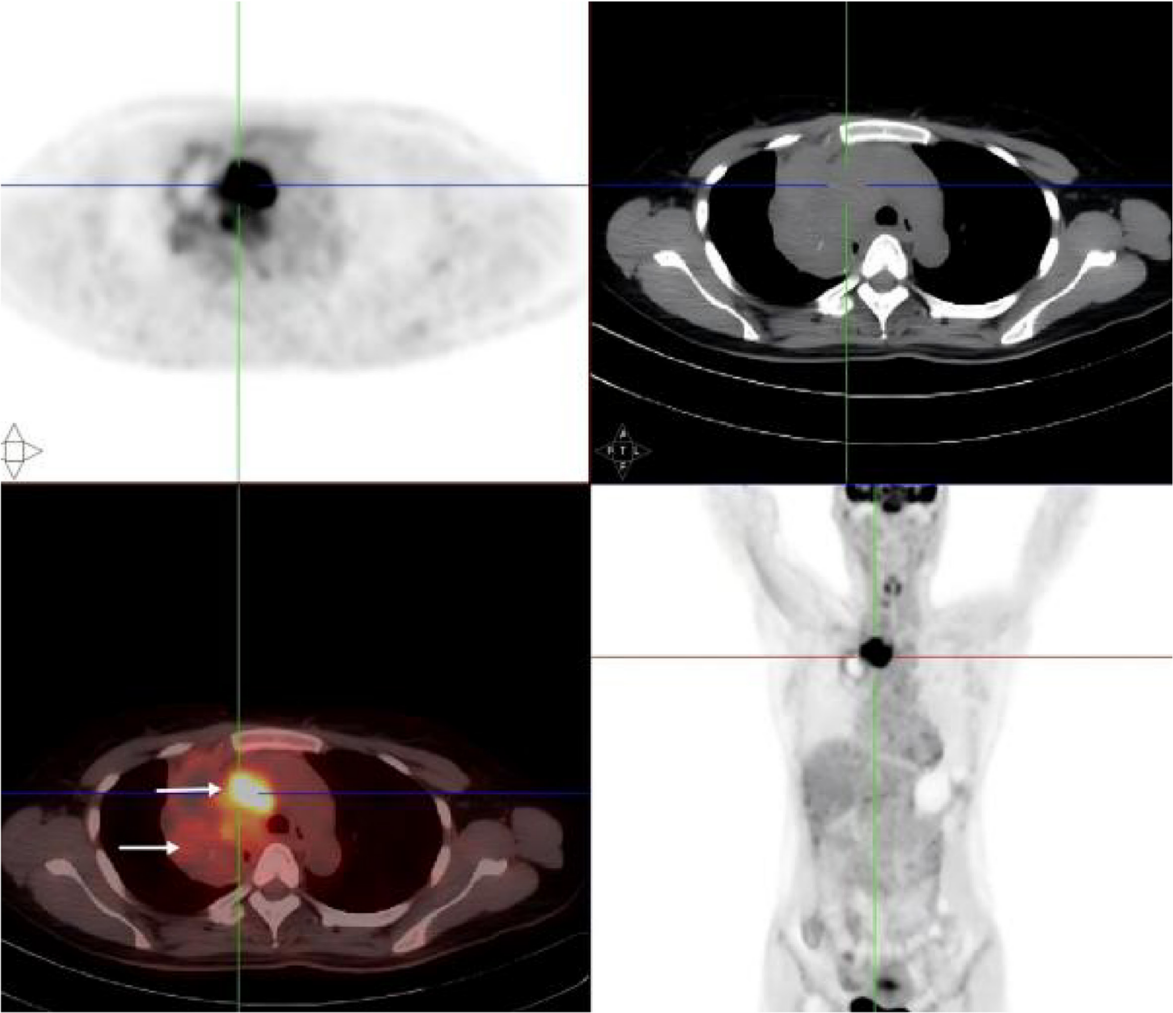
PET‐CT shows a mediastinal mass in the right superior lobe of the lung (→), with enlarged lymph nodes in the mediastinum (→) and right hilum of the lung, and increased FDG metabolism in the lesion.
